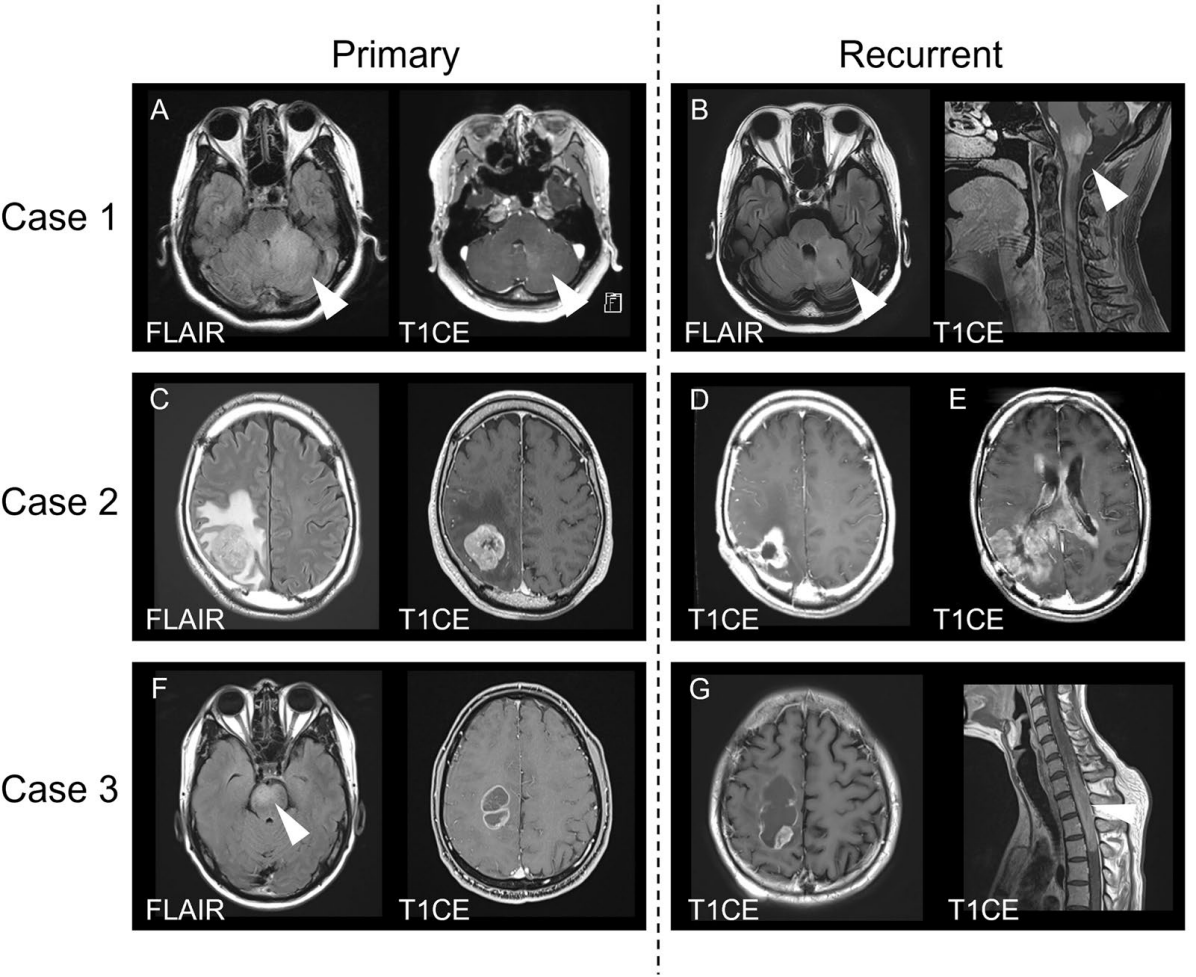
MRI of primary and recurrent tumors in the three cases. A FLAIR (left) and contrast-enhanced T1-weighted (right) images showing the primary tumor in Case 1. B FLAIR (left) and contrast-enhanced T1-weighted (right) images showing the recurrent tumor in Case 1. C FLAIR (left) and contrast-enhanced T1-weighted (right) images showing the primary tumor in Case 2. D Contrast-enhanced T1-weighted image showing the first recurrent tumor in Case 2. E Contrast-enhanced T1-weighted image showing the second recurrent tumor in Case 2. F FLAIR (left) and contrast-enhanced T1-weighted (right) images showing the primary tumor in Case 3. G Contrast-enhanced T1-weighted images showing a recurrent intracranial tumor (left) and cervical tumor (right) in Case 3. T1CE: contrast-enhanced T1-weighted imaging
